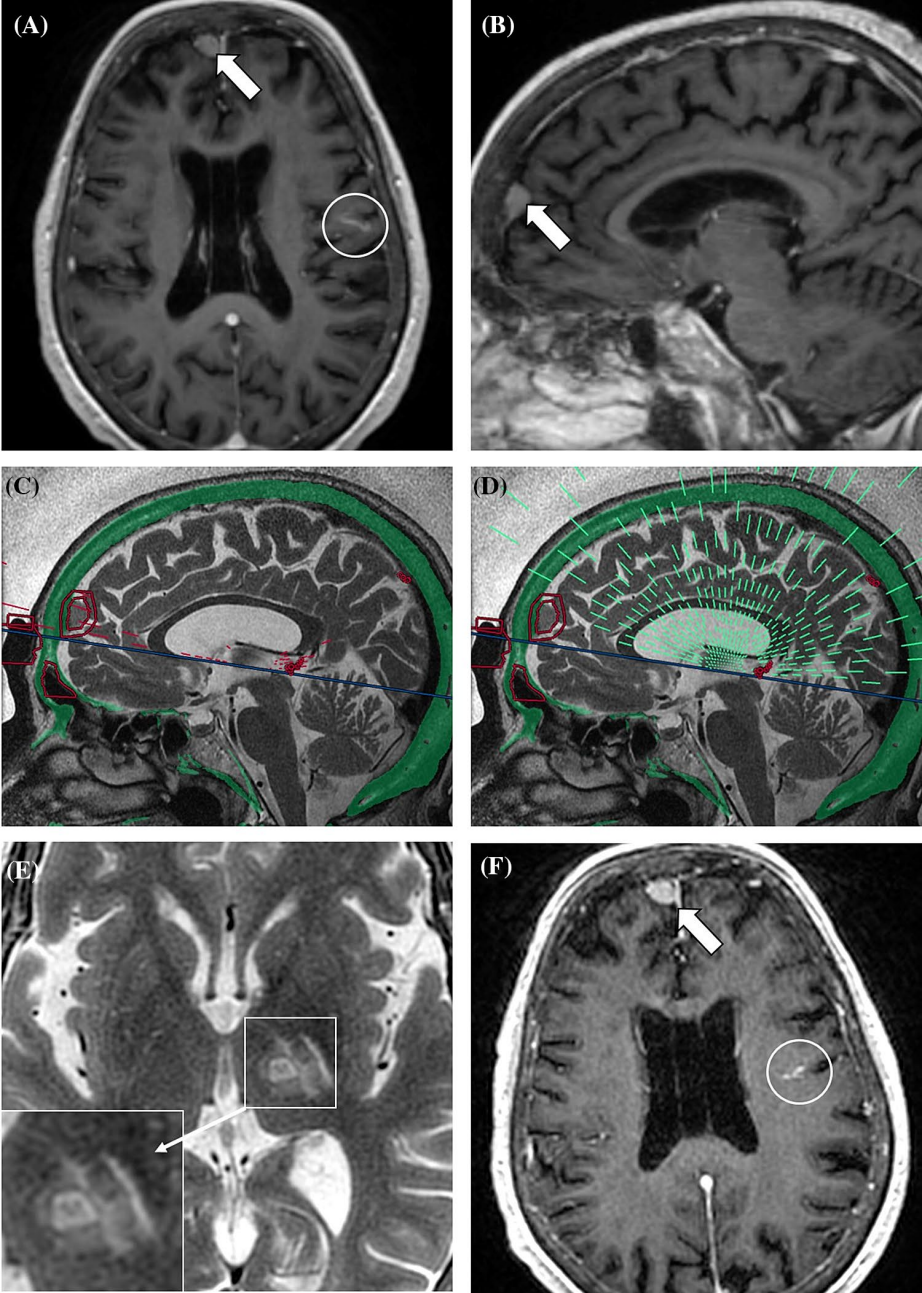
81-year-old woman with essential tremor. Preoperative axial (A) and sagittal (B) multiplanar reconstructions (3 mm thick, average algorithm) from post-contrast T1w 3D BRAVO demonstrate a 11 mm extra-axial lesion compatible with meningioma (arrows). A developmental venous anomaly in the left fronto-parietal region is also present (white circle). Sagittal intraoperative T2-weighted FRFSE images show no-pass regions (red circles) with blocked [(C) red lines] and active [(D) green lines] elements. A total of 13 additional elements (11.6% of all blocked elements) were turned off due to the presence of the meningioma. 48-h follow-up axial T2-weighted FRFSE sequence (E) shows the FUS-placed lesion in the left nucleus ventralis intermedius. Post-contrast (F) image demonstrates no changes in the meningioma (arrow) or developmental venous anomaly (white circle).
